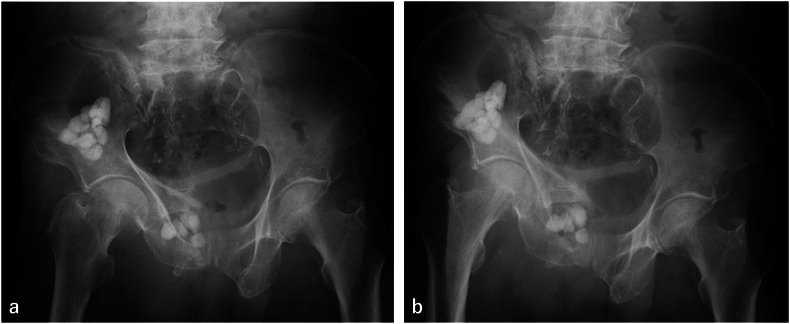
Plain X-ray photograph of the pelvis under push-pull test (a) The right lower limb is pulled caudally and the left lower limb is pulled cranially. (b) Pulled to the opposite side of (a). There is no obvious instability.
